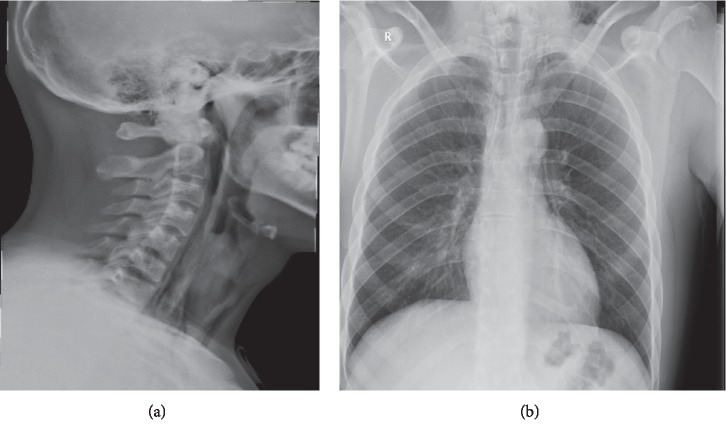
Lateral neck (a) and posteroanterior chest (b) X-rays at time of presentation showing air in neck spaces and chest has minimal air around the trachea but no pneumothorax seen and lung fields are clear.
